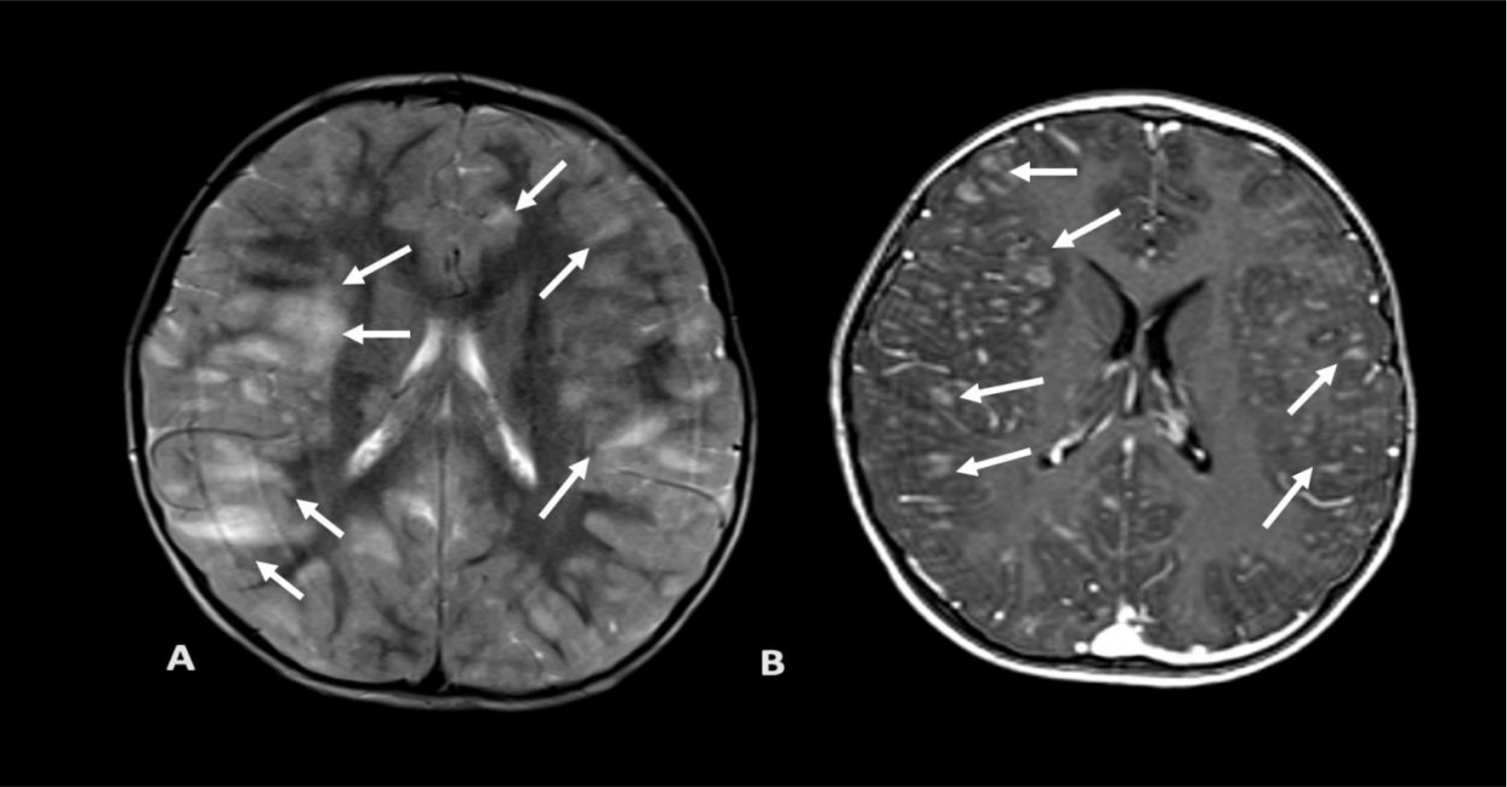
MRI brain images of P11 showing extensive supratentorial cortical and subcortical parenchymal damage with gliosis and white matter volume loss. Axial T2 weighted images (A), axial post-contrast T1 weighted-images (B) show uncountable enhancing lesions in the grey and white matter of all the cerebral hemispheres as well as infratentorial compartments (not shown). MRI – magnetic resonance imaging
